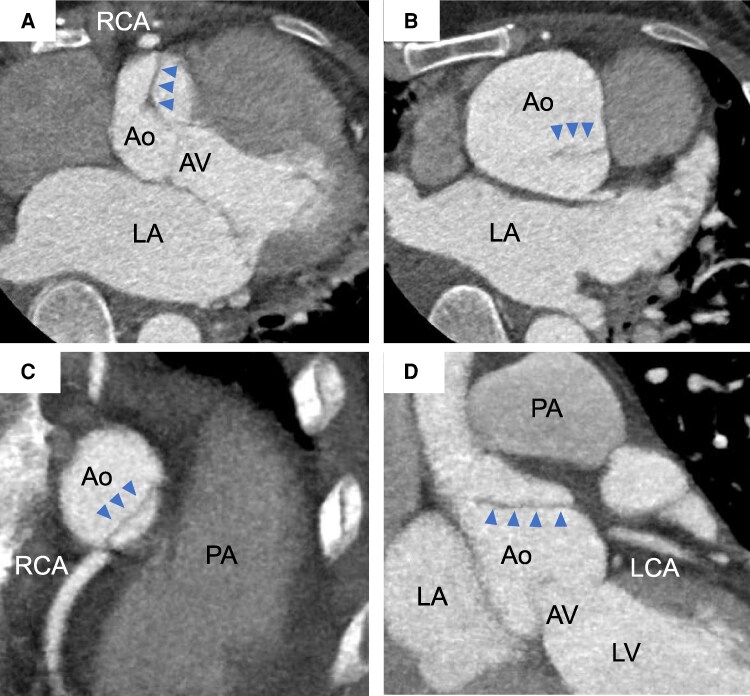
Cardiac-gated computed tomography. Cardiac-gated computed tomography showed aortic dissection (arrowhead) on the ascending aorta in axial view (*A* and *B*), which was extending to near the ostia of both the right and left coronary arteries in sagittal view (*C* and *D*). Ao, aorta; AV, aortic valve; LA, left atrium; LCA, left coronary artery; LV, left ventricle; PA, pulmonary artery; RA, right atrium; RCA, right coronary artery; RV, right ventricle.
